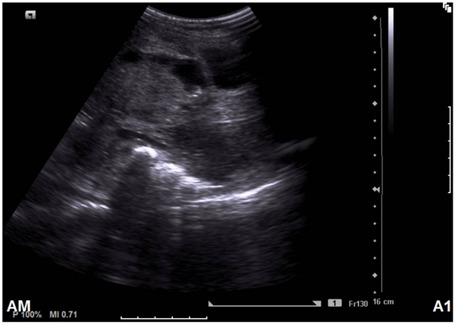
Radiographic image of Case 2. Pelvic ultrasound  shows hypoechoic predominantly endoluminal hetergenous  mass measuring 5×4.5×4.8 cm distending the upper vagina.
